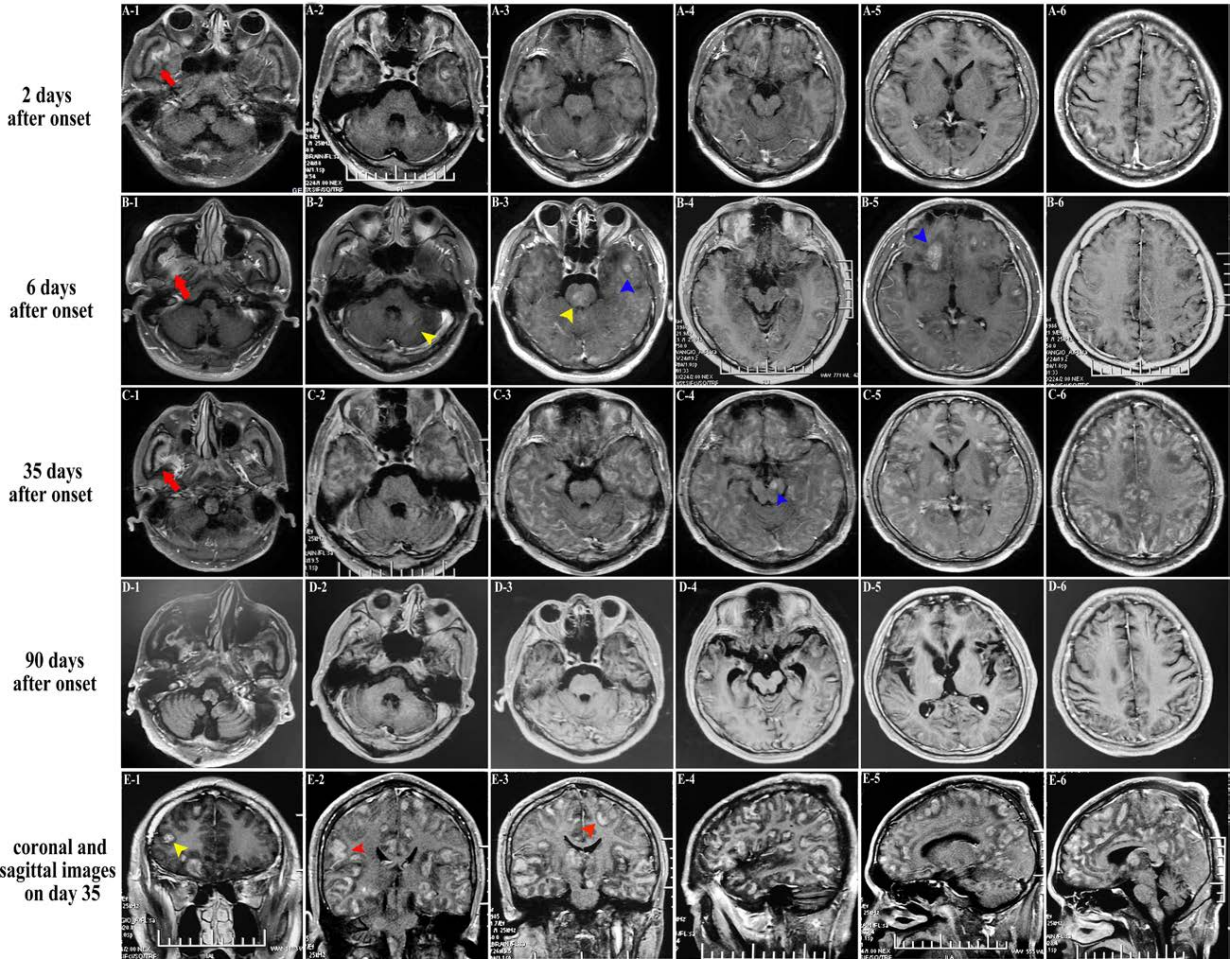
T1-weighted post-contrast axial imaging at different times after onset. Second day (A1‐A6); 6th day (B1‐B6); 35th day (C1‐C6); 90th day (D1‐D6); 18th months (E1‐E6); Various forms of enhancement including gyri-like (red arrowhead in E2, E3), patchy (yellow arrowhead in E1, B2, B3) and nodular (blue arrowhead in B3, B5, C4) pattern with meningeal enhanced were displayed at 6th and 35th day. MR images on 90th day showed a decrease in degree and number of the lesions. Patchy hyperintense signals in right temporalis muscle and outer pterygium muscle suggested temporomandibular space infection (red arrow in A1, B1, C1).
